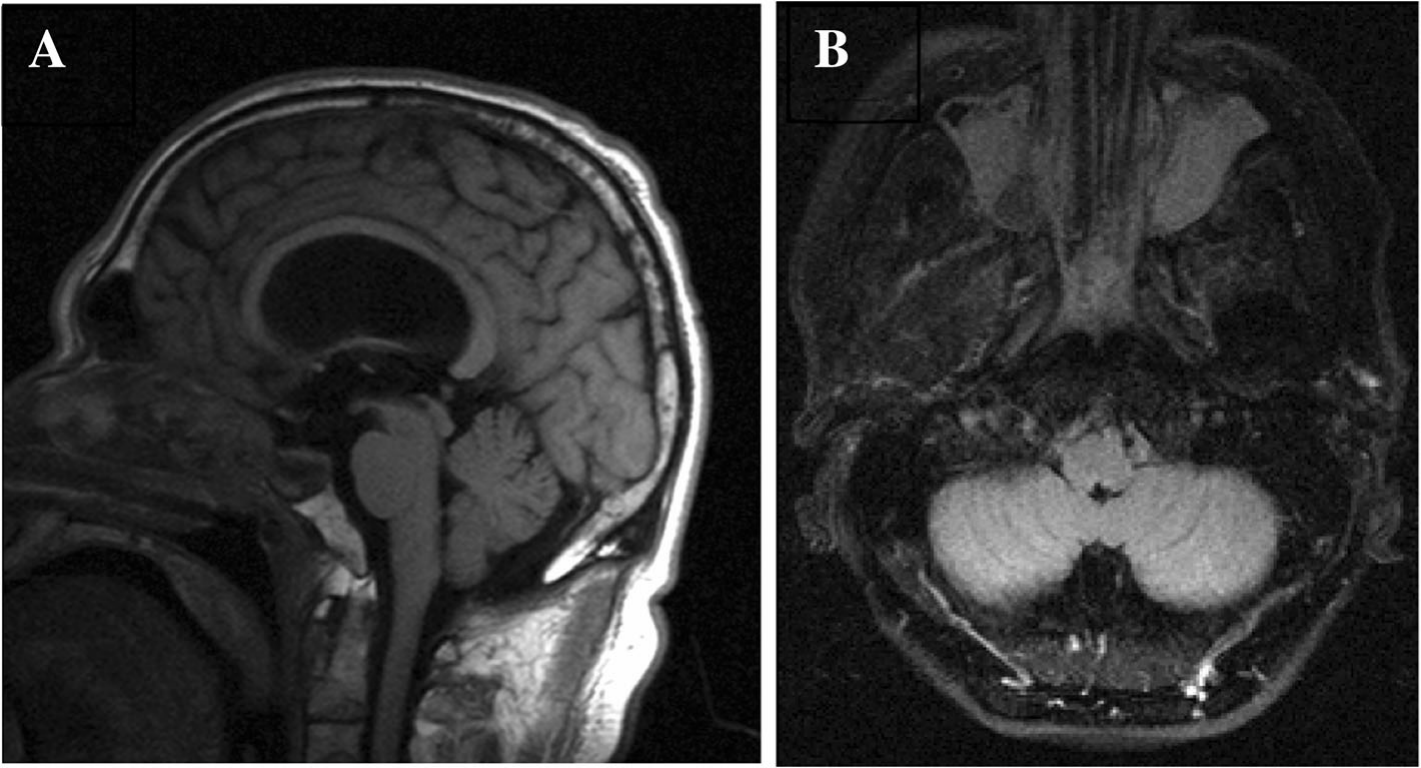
Magnetic resonance imaging without contrast, postoperative images. a Sagittal and b axial magnetic resonance imaging postoperative images showed no evidence of residual meningoceles
